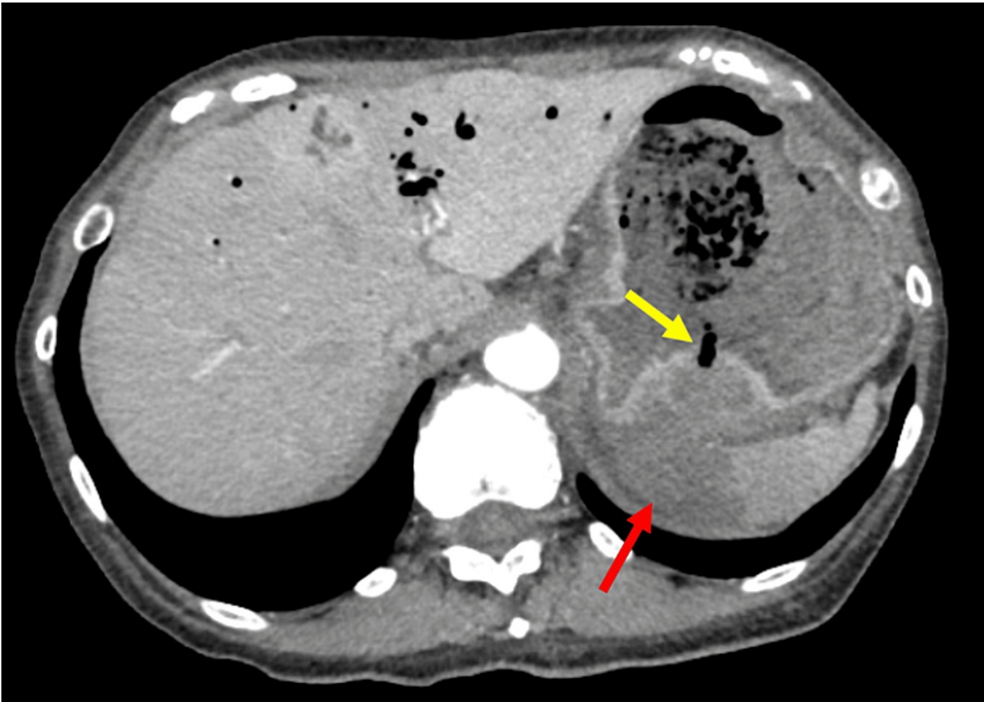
CT findings upon arrival Discontinuity in the posterior gastric wall adjacent to the hematoma around the splenic artery aneurysm (yellow arrow) and evidence of splenic infarction (red arrow) CT: computed tomography
